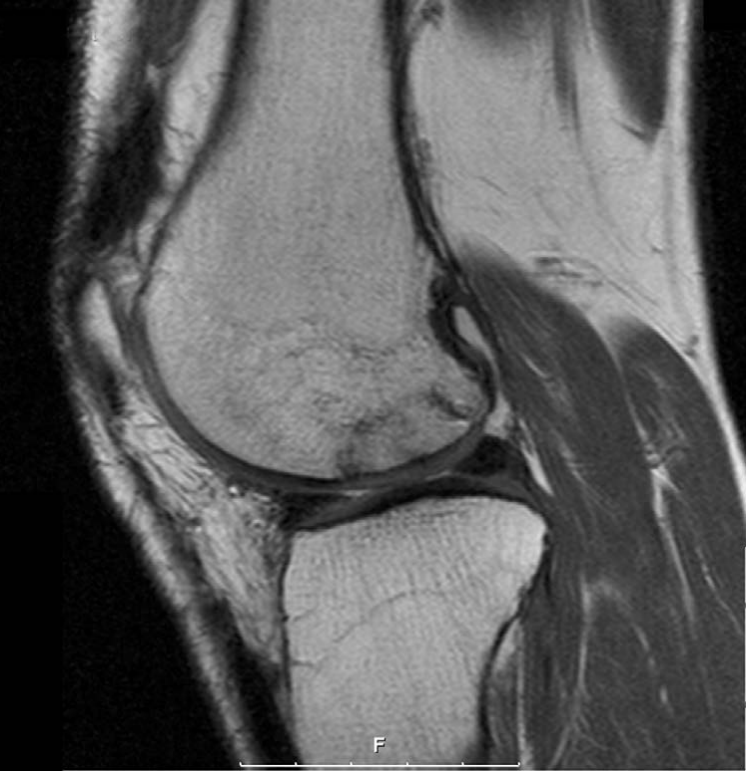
Magnetic resonance image after the large osteochondral autogenous transplantation system technique. Magnetic resonance imaging scan of Patient 23, 59 months after the large osteochondral autogenous transplantation system and cancellous bone grafting.
